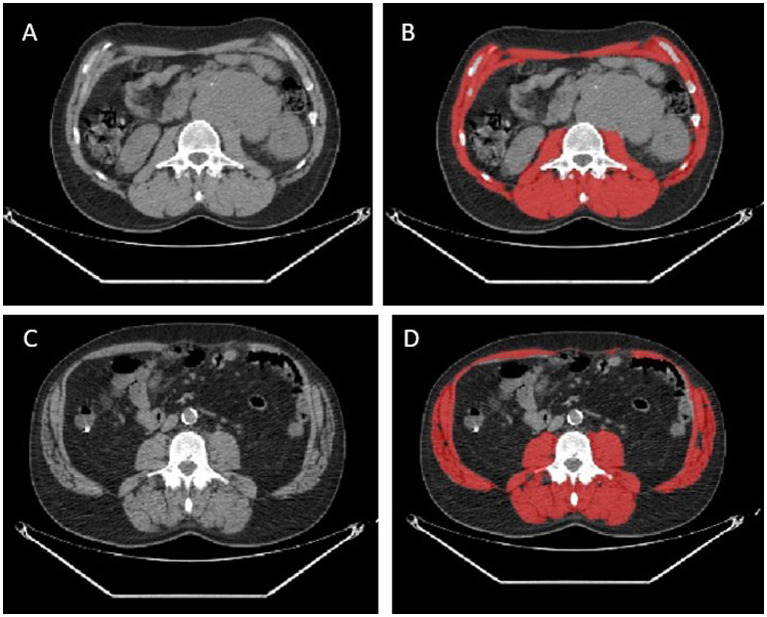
Two representative cases of patients with no sarcopenia detected by CT images (A,B) and sarcopenia (C,D). In red the measurement of skeletal muscle area by the software.
